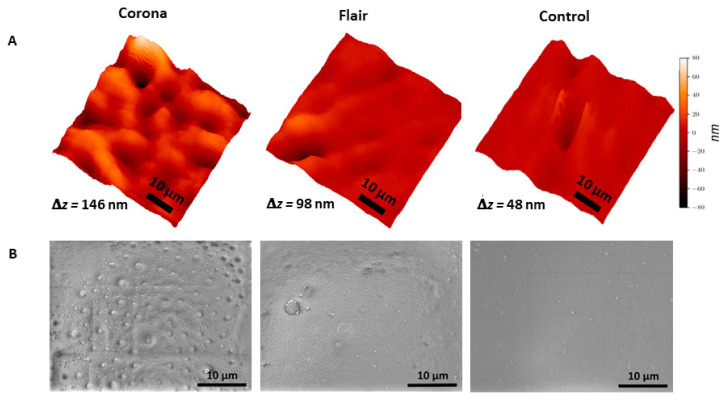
X-ray ptychography (A) and SEM (B) images of plasma-treated and control PET films. Δ*z* is the peak-to-valley difference.
